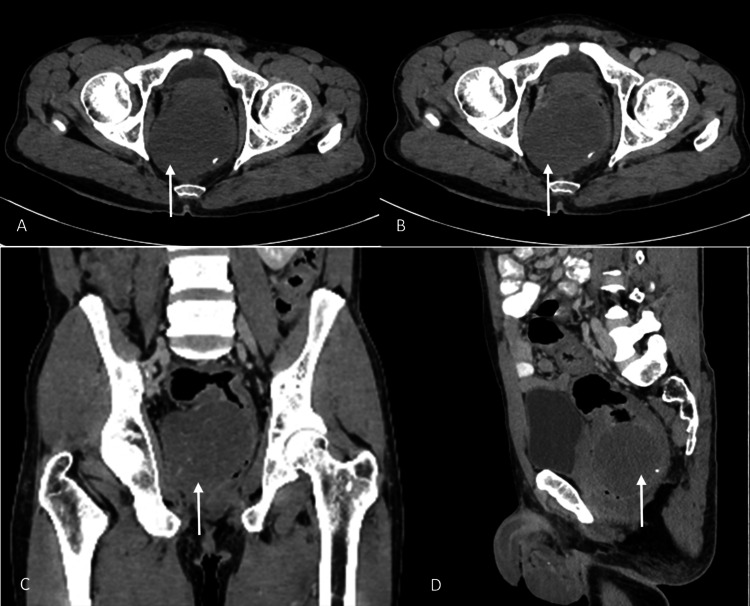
NCCT and CECT scans of the abdomen and pelvis (A) NCCT axial view; (B) axial view; (C) coronal view; and (D) sagittal view of the CECT scans of the abdomen and pelvis show a well-defined hypodense, enhancing lesion with a small peripheral calcification in the rectum (white arrows). The lesion caused a significant luminal narrowing of the rectum. NCCT: non-contrast CT, CECT: contrast-enhanced CT
